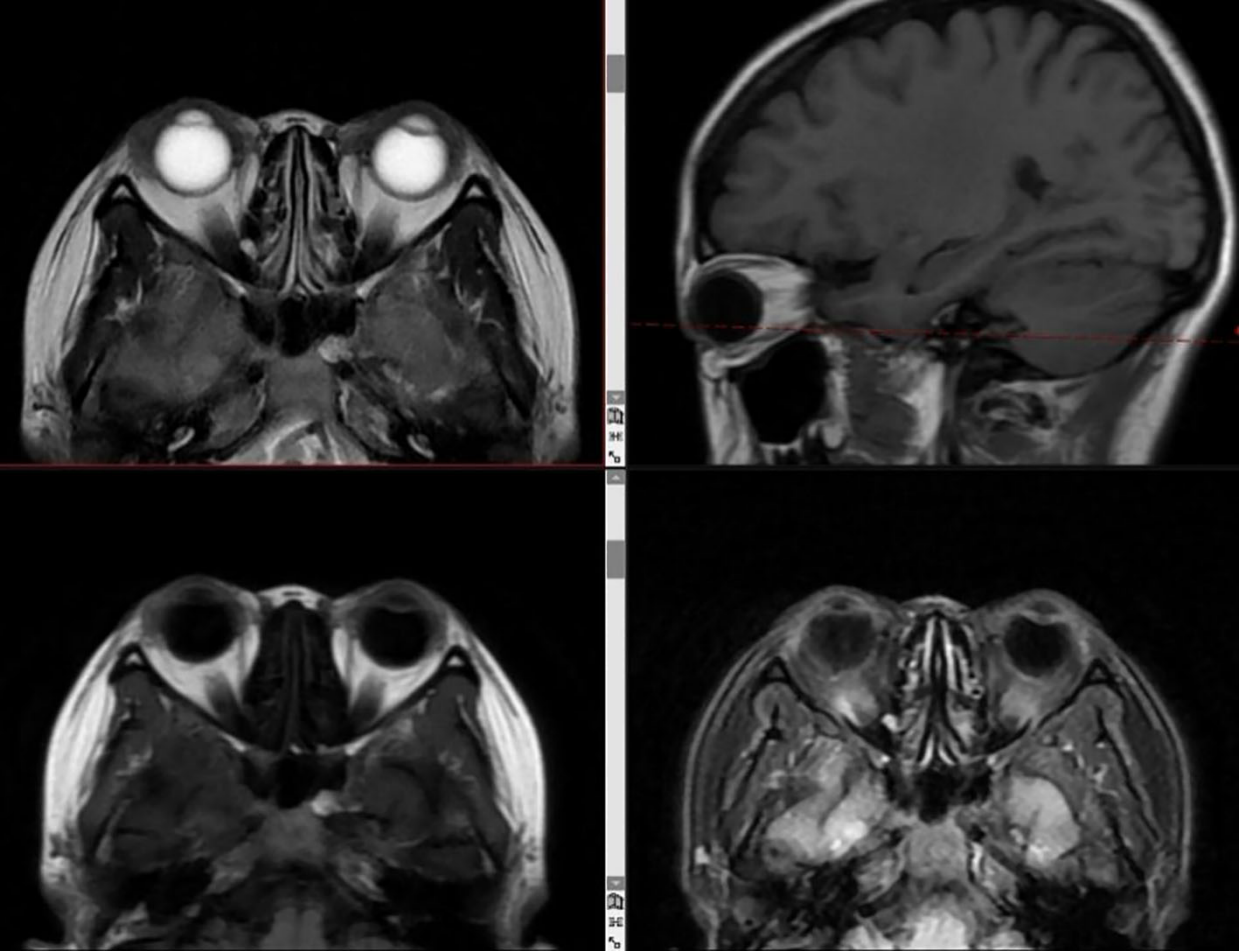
MRI shows complete resolution of ocular and intracranial lesions after treatment. T1WI, T2WI, T2Flair, and DWI images show no abnormal signals in the eye region; no abnormal enhancement was observed after contrast administration. All brain structures appear normal.
